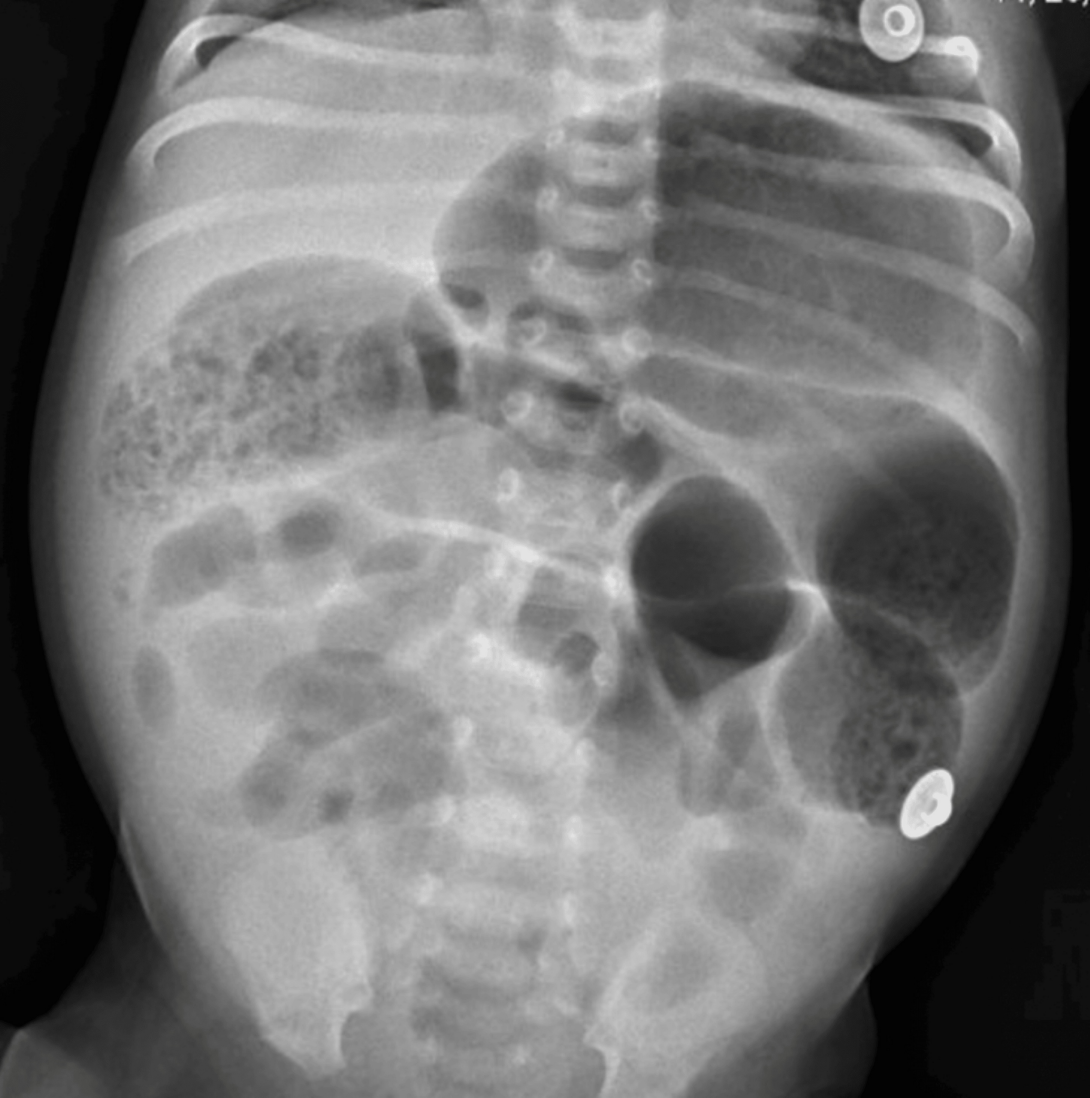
Abdominal radiograph at initial presentation Abdominal radiograph demonstrating findings suggestive of bowel ischemia or small bowel obstruction. The bowel pattern is irregular with gaseous distention of the stomach and distention of multiple loops of bowel with mottled lucencies. There is no portal venous gas.
